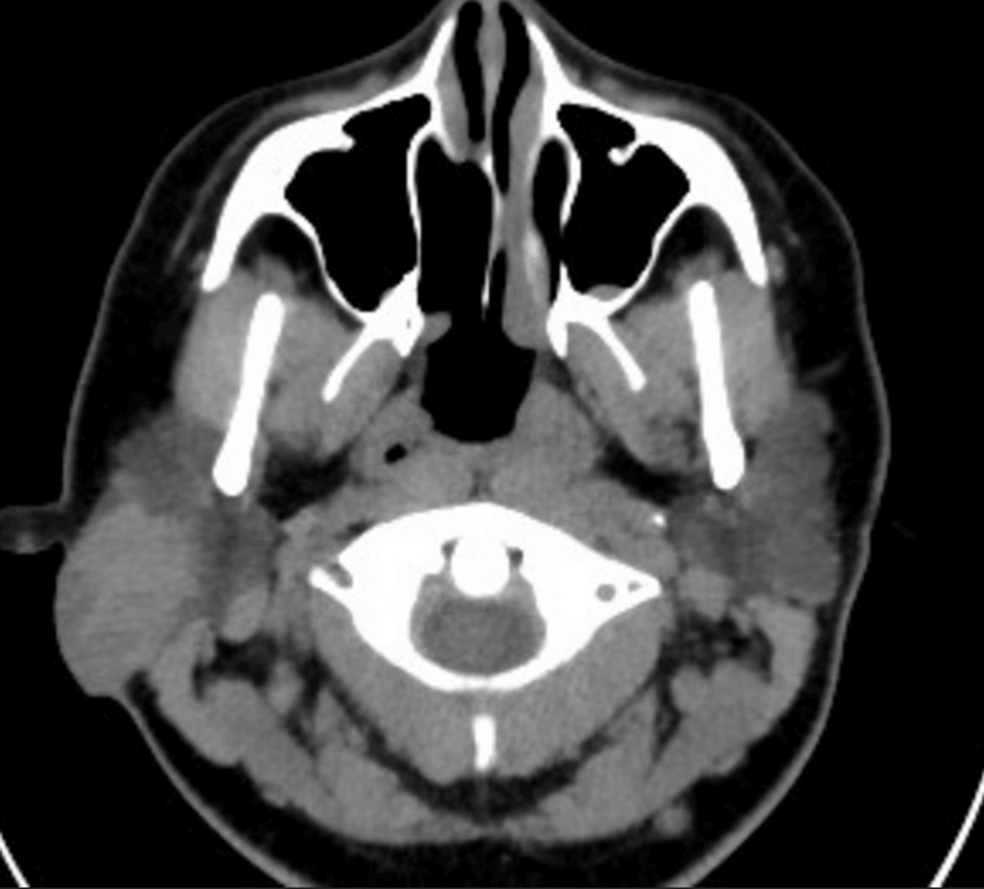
Contrast-enhanced computed tomography (axial image) An expansive lesion was identified located in the parotid gland in the superficial lobe, which was isodense to the muscle, had hypodense areas and smooth edges, and presented an extension to the subcutaneous cellular tissue.
